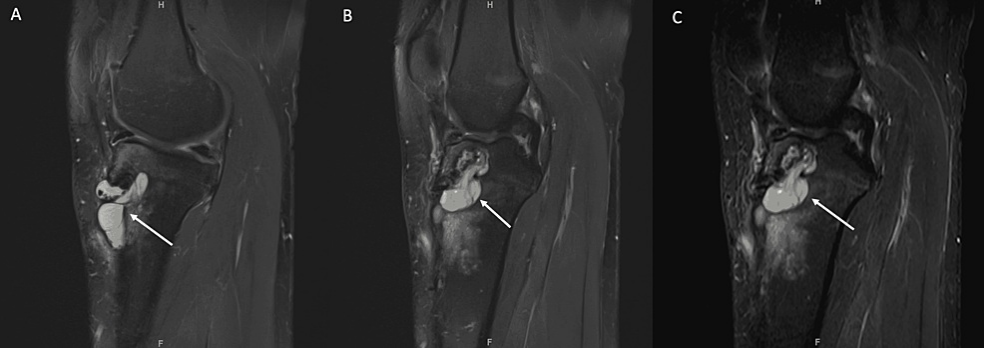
Sagittal knee MRI scans of the pretibial cyst showing its communication with the tibial tunnel. A) Sagittal knee MRI scan (proton density fat-saturated (PD FS)); B) sagittal knee MRI scan (PD FS); C) sagittal knee MRI scan (short T1 inversion recovery (STIR)).
